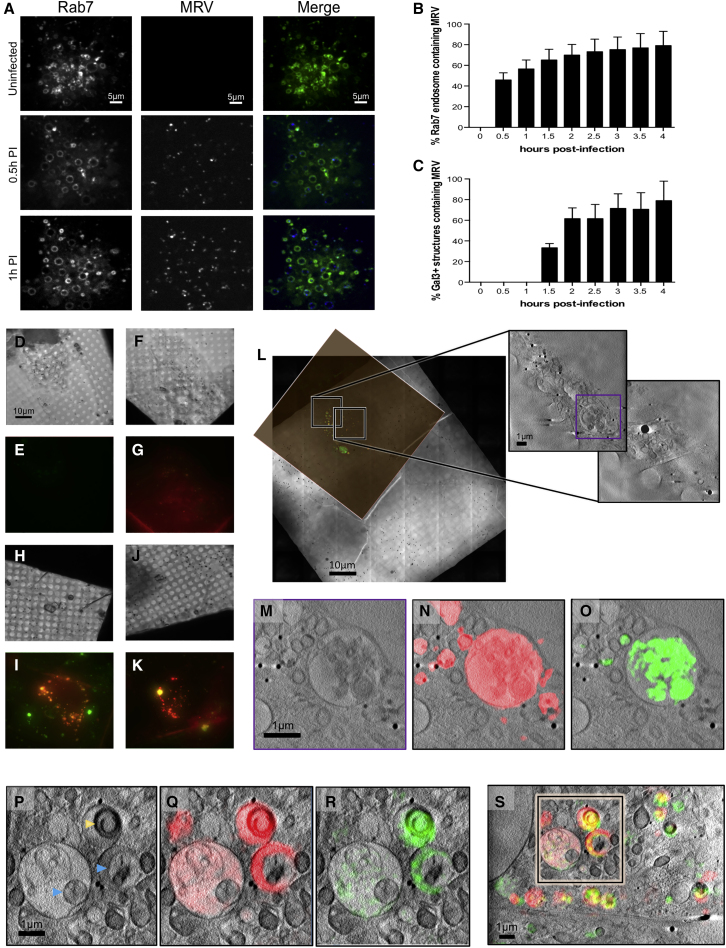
Tracking reovirus endosomal trafficking and escape (A) Confocal images of U2OS cells expressing late-endosome marker Rab7-eGFP infected with Alexa647-labeled reovirus at indicated times AI. (B and C) Mammalian reovirus (MRV) presence in late endosomes up to 4 h AI (B). Numbers of Gal3--positive endosomes containing MRV for the same period (C) (error bars indicate SD of n = 8 in B and n = 9 in C). (D–K) CryoSIM images of MRV labeled with Alexa488 in mCherry-Gal3 expressing cells showing the concentrations of Gal3 signal in distinct vesicles in red; brightfield and SIM slices respectively at: time 0 mock-uninfected (D and E); 1 h AI (F and G); 2 h AI (H and I); and 4 h AI (J and K). (L) Superposition of SIM processed data of a 4 h AI sample on the 2D X-ray mosaic of the same area and expansion of adjacent FOVs where two adjacent X-ray tomograms were collected and stitched. (M) Expanded region of interest (highlighted purple in L). (N and O) Same as (M) with Gal3 signal in red (N) and reovirus signal in green (O), respectively. (P–R) ROI from 2 h AI with either X-ray grayscale imaging alone or superposed wiith fuorescence signal: single z axis slice from the corresponding tomogram with Gal3 (red) and MRV (green) localization. Multi-vesicular bodies (MVBs) are clearly identifiable with distinct sub-compartments where Gal3 is concentrated (presumably the result of virus-carrying vesicles fusing with virus free late endosomes). Virus-induced carbon-rich substructures are marked by yellow arrows and vesicle areas within MVBs that remain impermeable to Gal3 marked by blue arrows. (S) Overview of the relevant 2 h AI region with both fluorescent signals overlaid.
